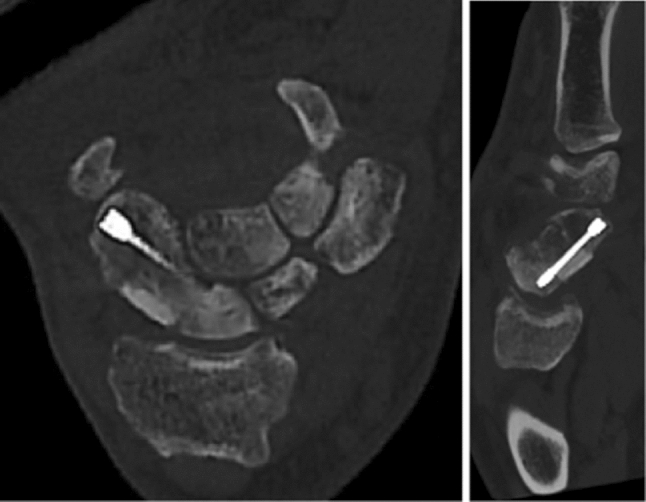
CBCT with Sander's reconstructions 6 weeks postoperatively
